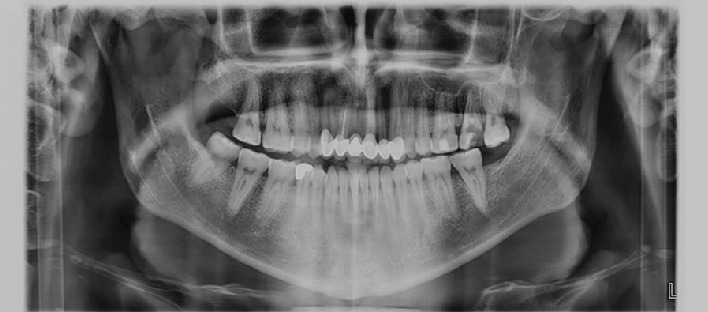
Orthopantomogram before treatment showed the upper left second molar opened with periapical radiolucency.
